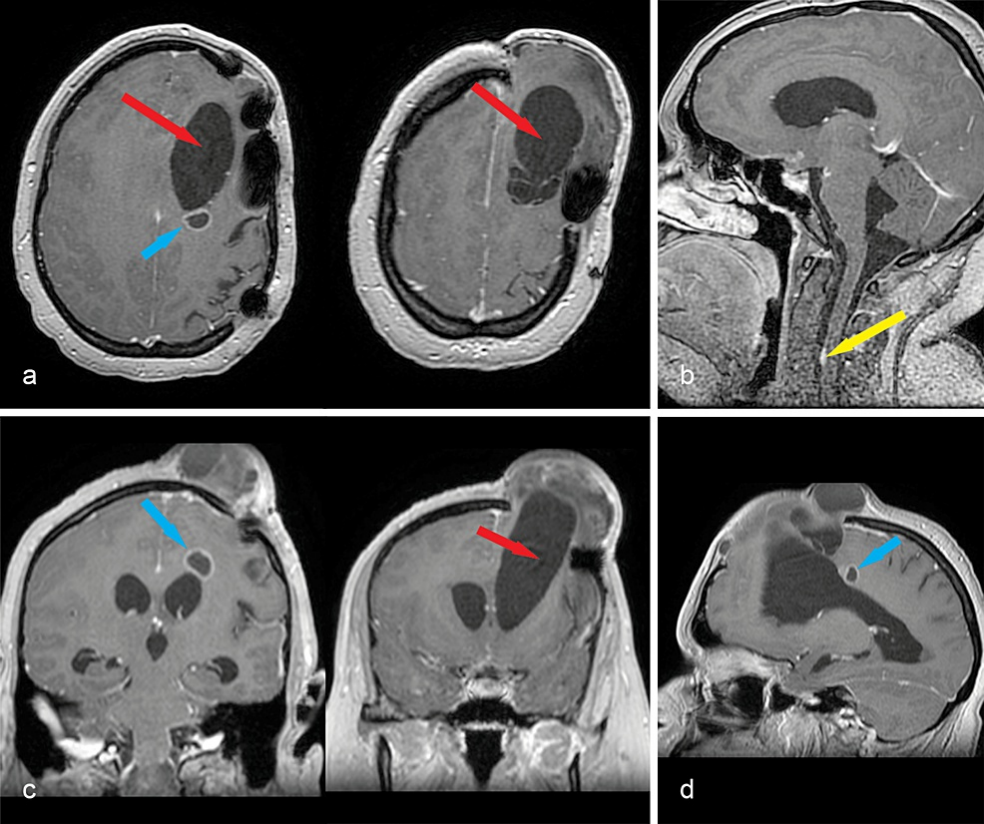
a, c, d: axial, coronal, and sagittal contrast-enhanced T1 MRI sequences depict the cystic mass (red arrow) causing retraction and dilation of the left frontal horn, with possible communication with the ventricle; the cystic lesion and the ventricular horn prolapsed through the bone defect; the ventricles are enlarged, with periventricular resorption halo; the second, parietal tumor is slightly enlarged (blue arrow); b: sagittal contrast-enhanced T1 sequence shows the new dural-based lesion next to the C3 vertebra (yellow arrow)
